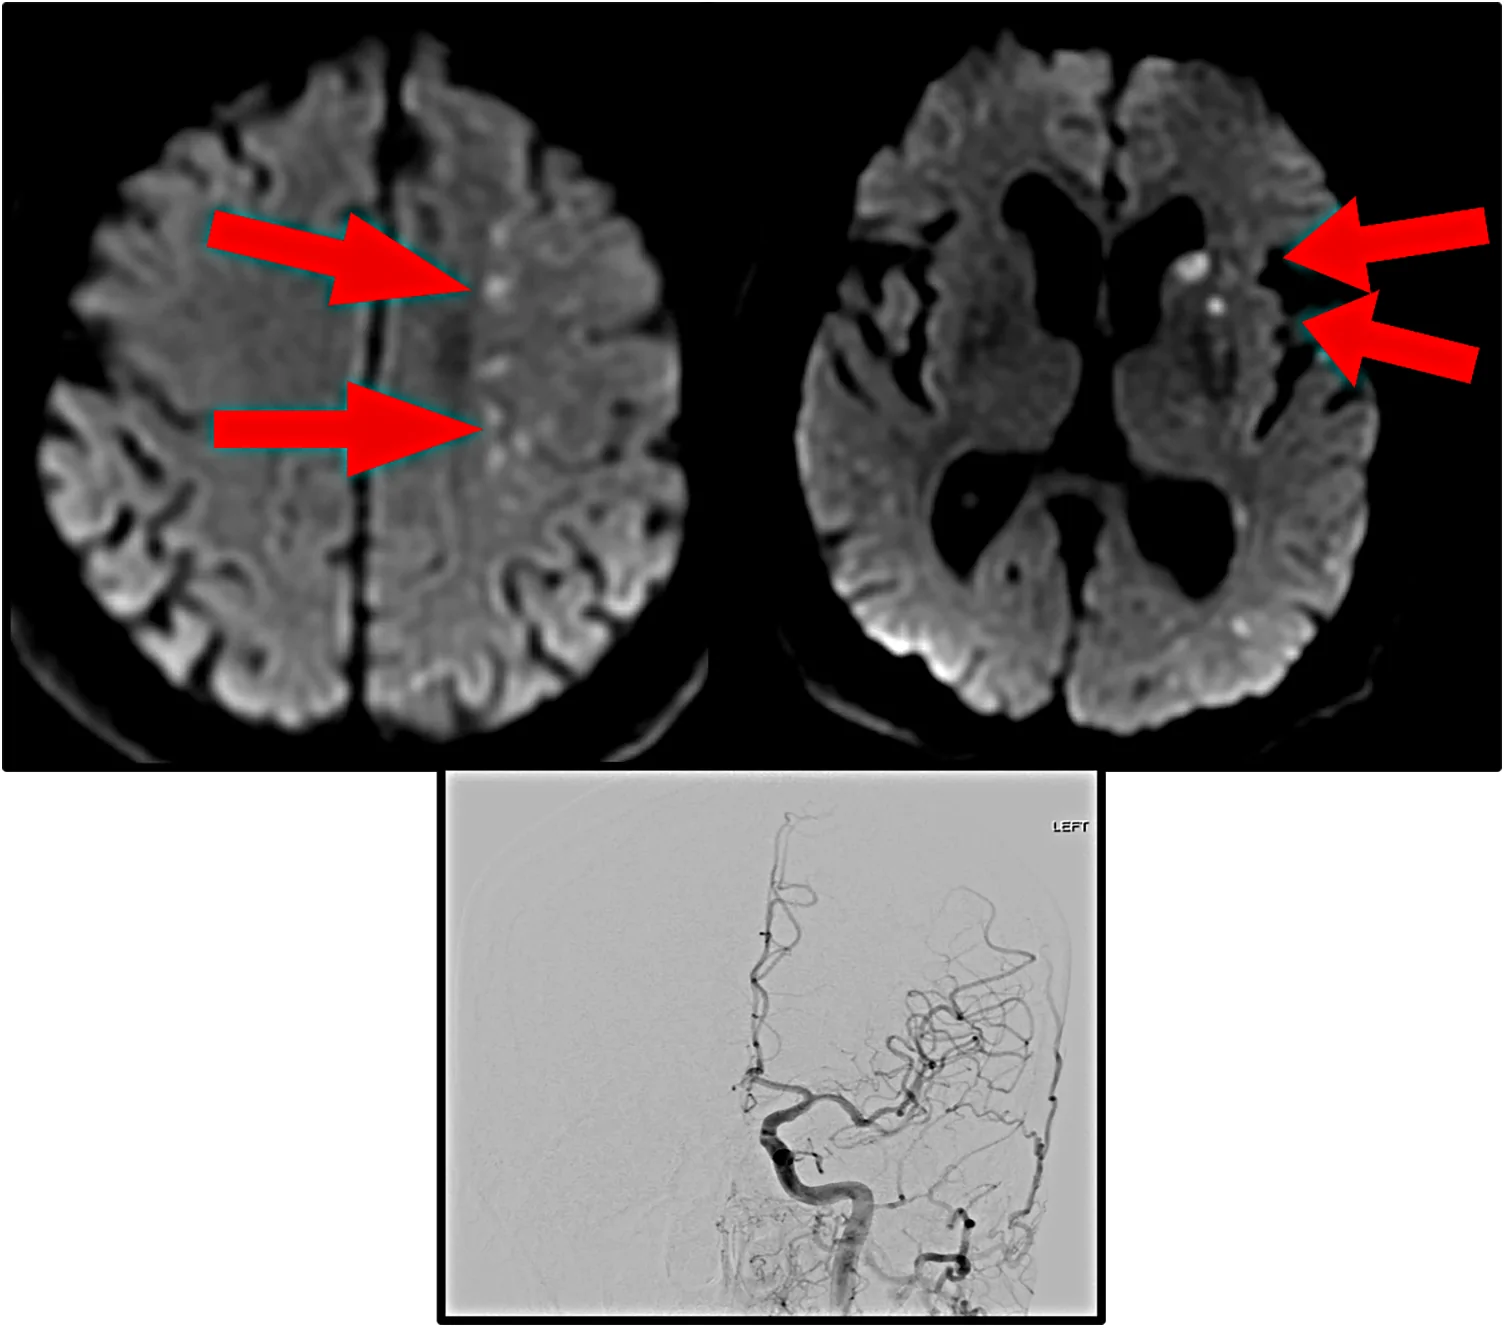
Top: MRI brain with diffusion restriction in the left hemisphere due to a heavily calcified atheroembolism from the left ICA stenosis. Bottom: Left AP Townes intracranial angiography with anterograde filling of the left MCA and left ACA branches.
